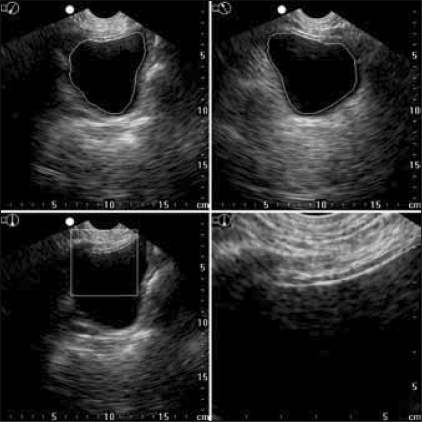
Trans-abdominal ultrasound images of the bladder at 3.7 MHz using the BVM6500 (Verathon Medical, Bothell, Washington State, USA). The software automatically identifies the bladder (white line) and the bladder wall (yellow line)
